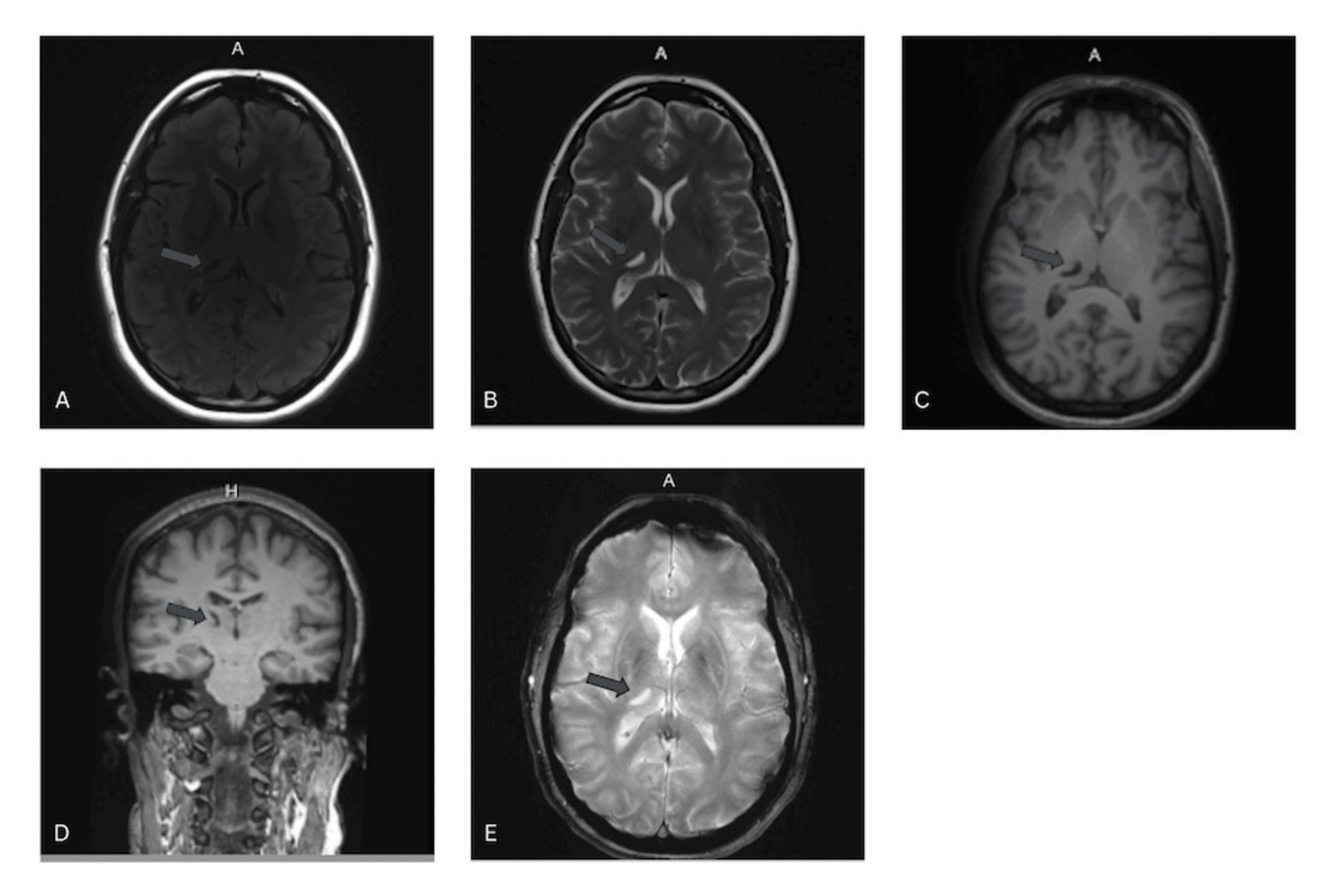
2022 MRI Brain Imaging (A) Axial T2 fluid-attenuated inversion recovery (FLAIR)-weighted magnetic resonance image; (B) Axial T2-weighted sequence; (C) Axial T1-weighted sequence; (D) Sagittal T1-weighted sequence; (E) Axial susceptibility heme-weighted sequence.
